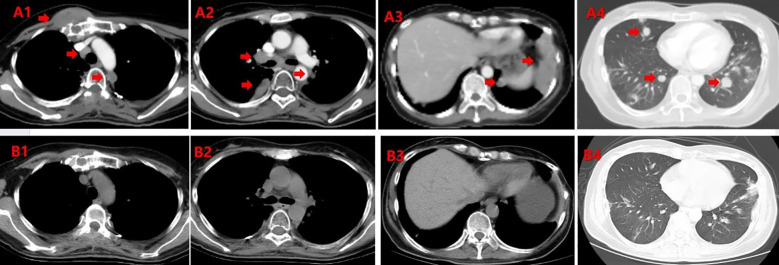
Comparative imaging of Patient 5 before and 1.5 months after HT-WLI-SIB. Pre-RT CT images (A1–A4) and post-RT CT images (B1–B4) show: (B1) Complete response of mediastinal lymph nodes and left pleural lesions; partial response of sternal metastasis. (B2) Complete response of hilar lymph nodes; near-complete response of right pleural lesion. (B3) Partial response of left pleural lesion; complete response of left pulmonary metastasis. (B4) Complete response of pulmonary metastases.
